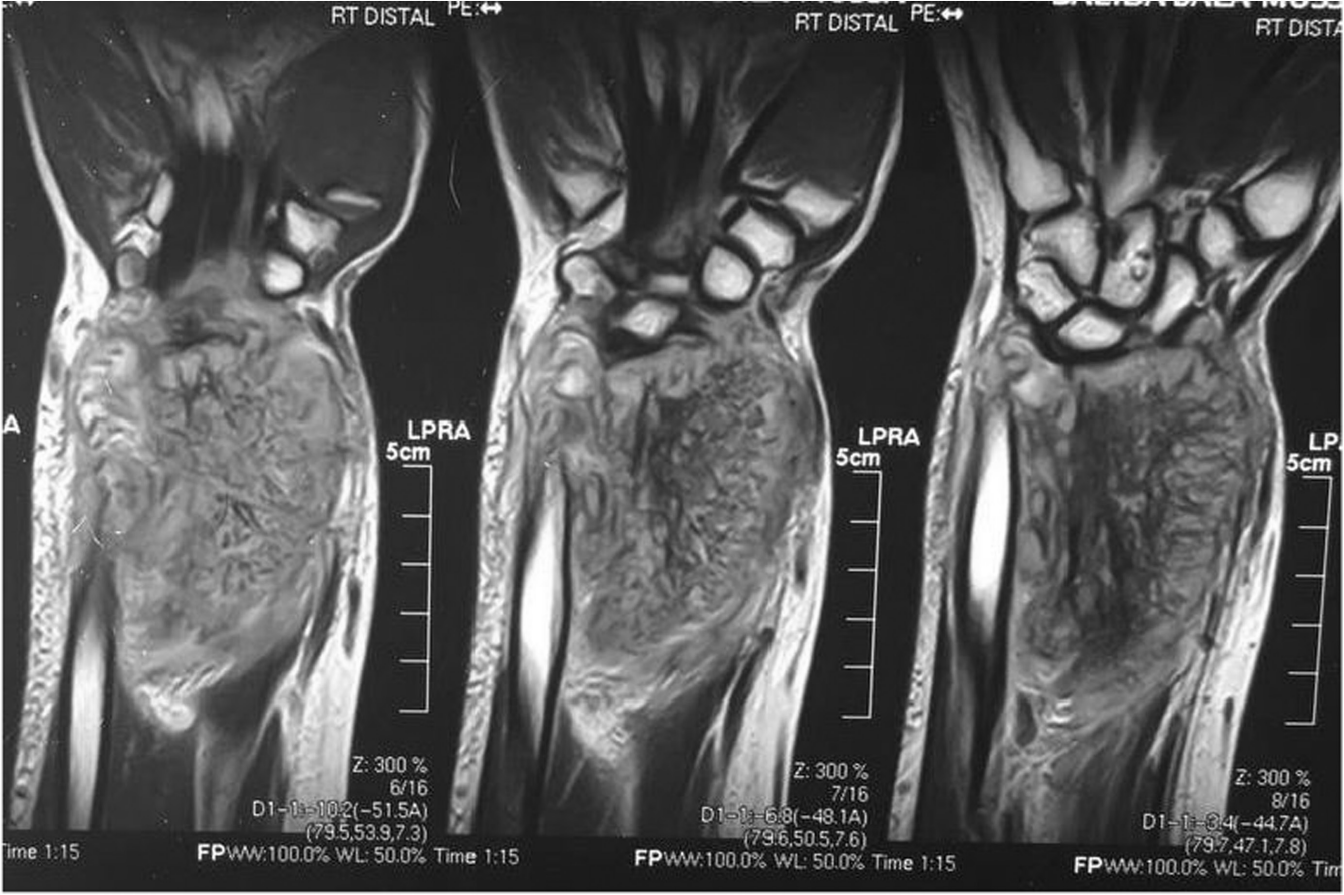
Preoperative MRI coronal view of Rt forearm shows right distal forearm swelling (enhancement) incasing distal neurovascular bundle, pretumor edema, and no skip lesions.
